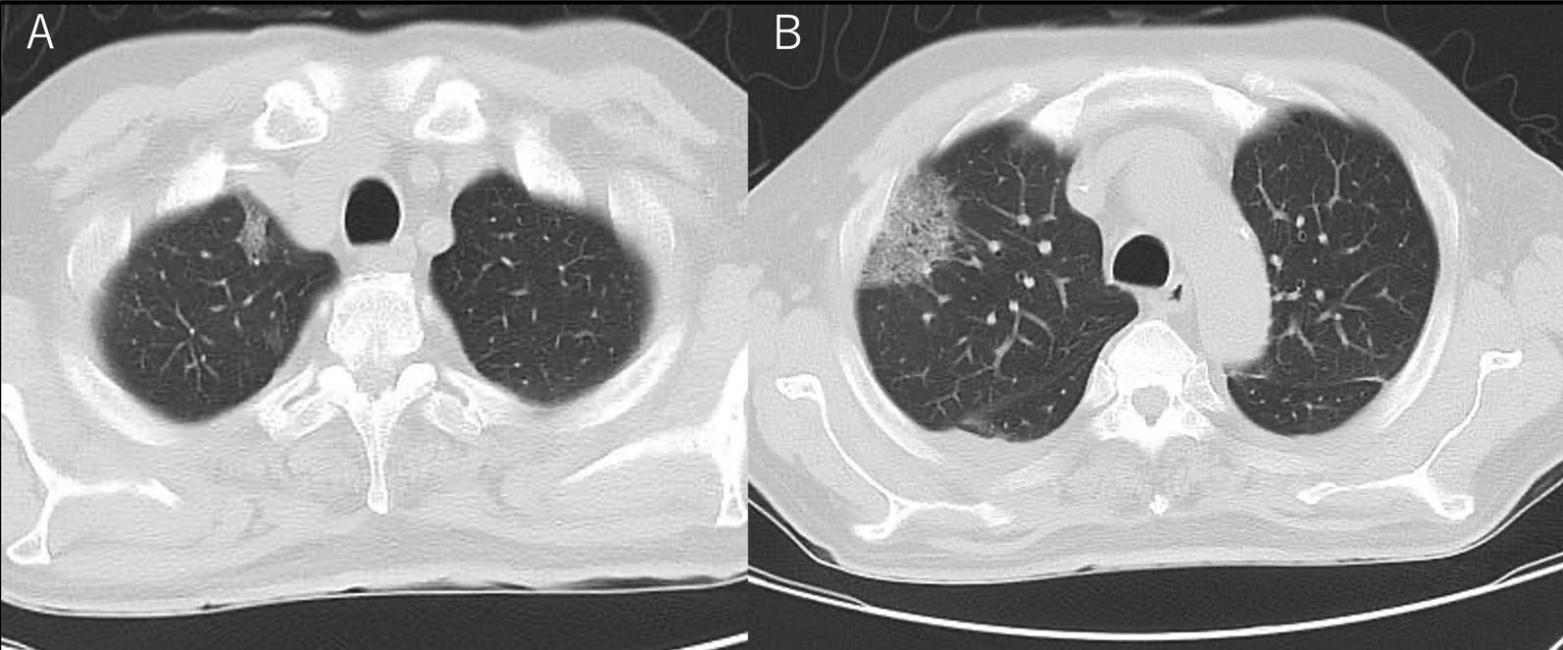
Chest computed tomography scan obtained on the second day after admission. A The appearance of a new GGO in the right upper lobe. B The existing GGO appears enlarged and shows thickening of the interlobular and intralobular septal walls. *GGO* ground-glass opacity
